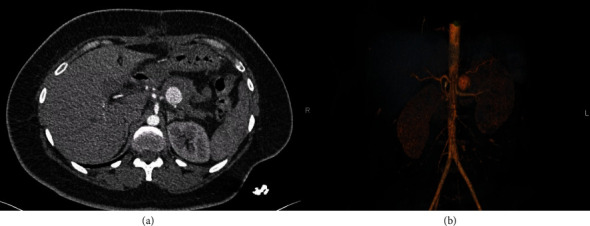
Abdominal CT angiography showing splenic artery pseudoaneurysm after central pancreatectomy. (a) Cross-sectional view. (b) 3D reconstruction. CT, computer tomography.
